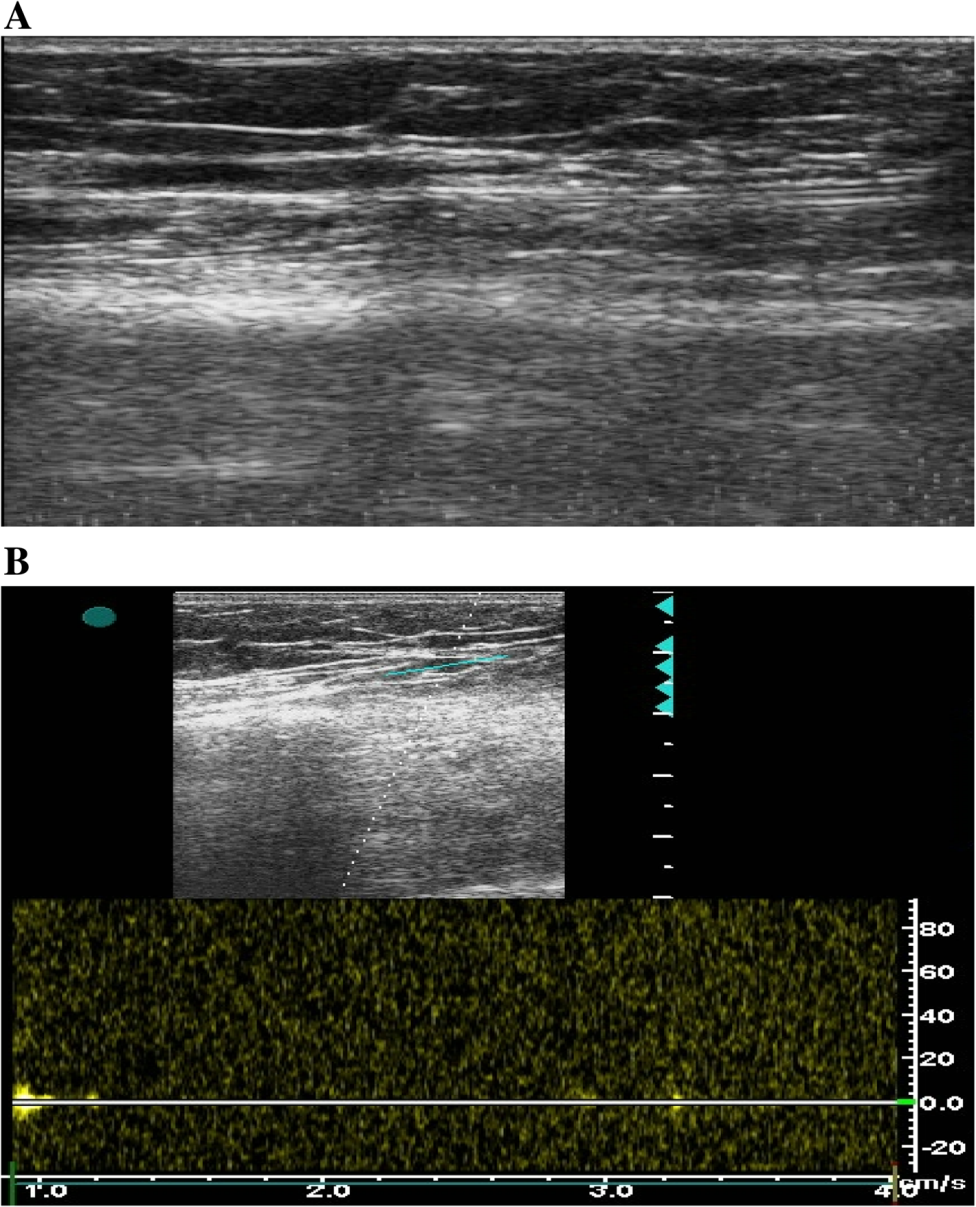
Duplex ultrasonography of an occluded RA after TR coronary catheterization. a B-mode showing total occlusion of the RA with thrombosis distal to the site of occlusion. b Spectral analysis showing no flow distal to the site of occlusion
